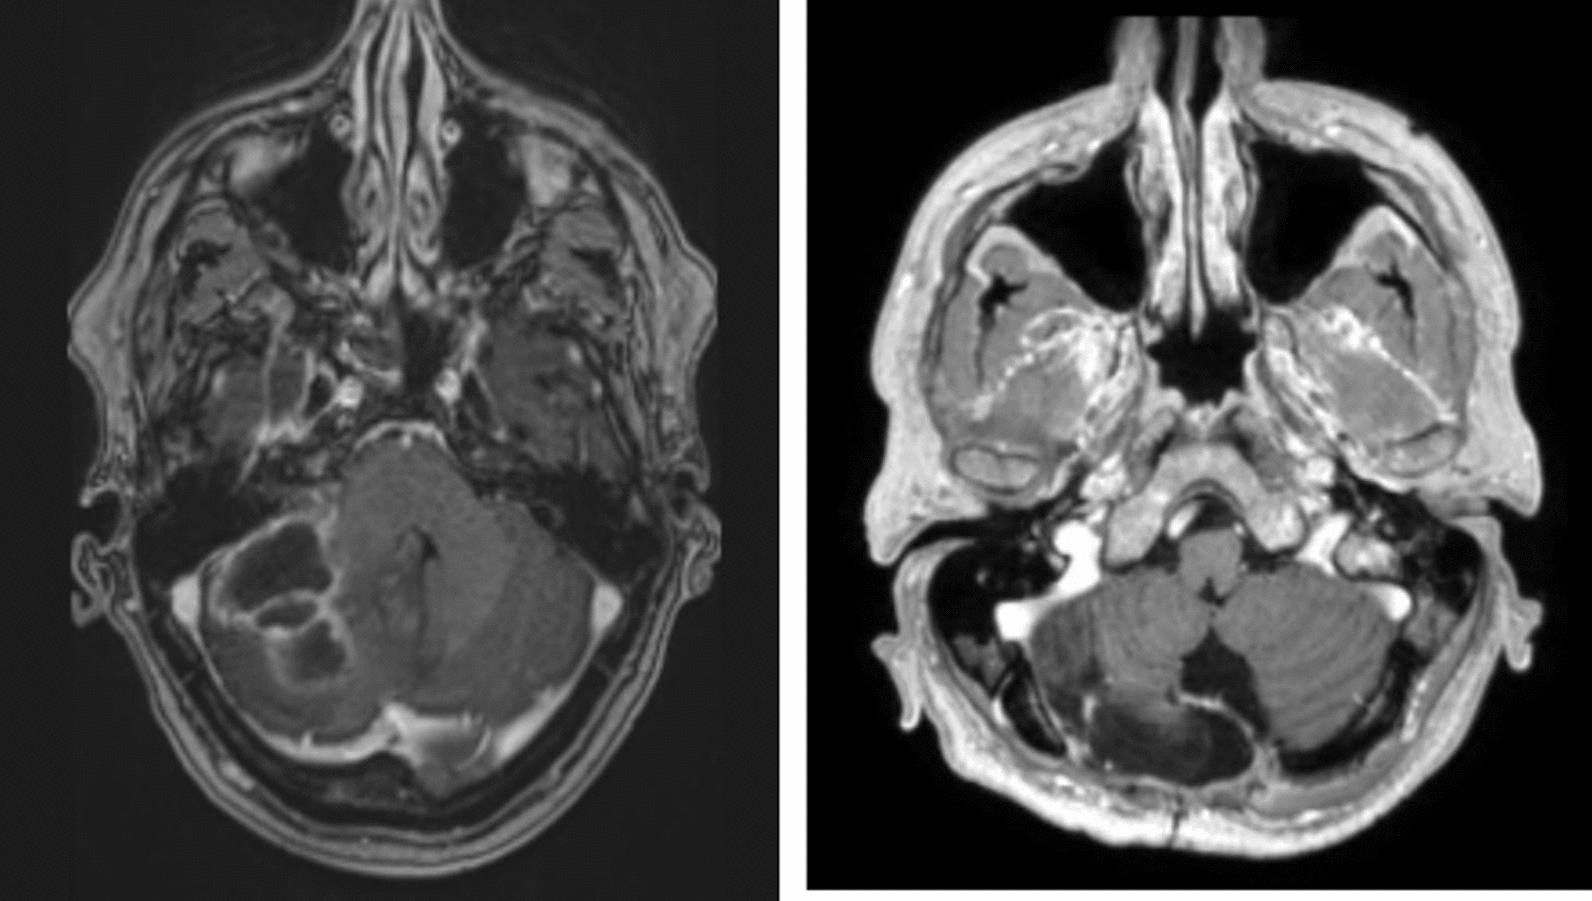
The initial magnetic resonance imaging with gadolinium enhancement revealed a lesion in the right cerebellar hemisphere, the follow-up magnetic resonance imaging with gadolinium enhancement after 6 months and after completion of antibiotic therapy showed no signs of residual lesions
